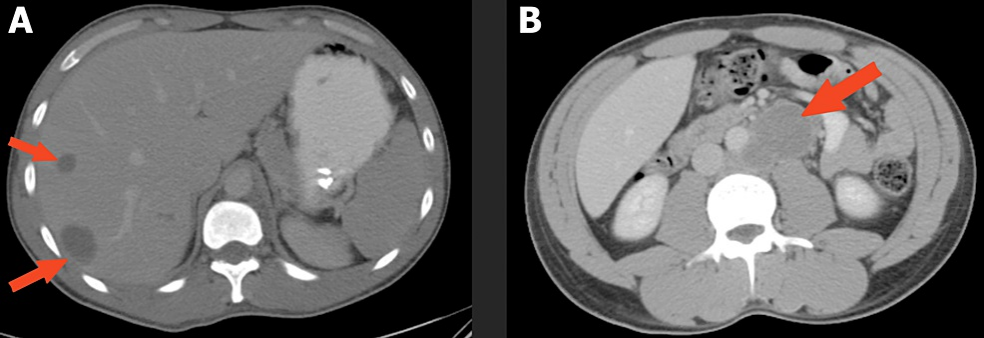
(A) CT abdomen with liver metastases. (B) CT with bulky retroperitoneal lymph nodes. CT, computerized tomography
